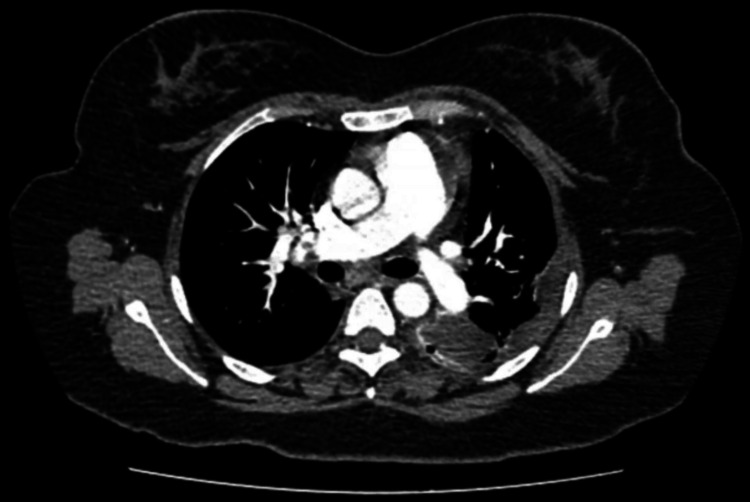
Contrast-enhanced computed tomography pulmonary angiography (CTPA) Contrast-enhanced computed tomography pulmonary angiography (CTPA) demonstrating extensive thrombus within the right main pulmonary artery and its lobar branches, as well as additional thrombi in the left pulmonary artery, consistent with massive pulmonary embolism.
